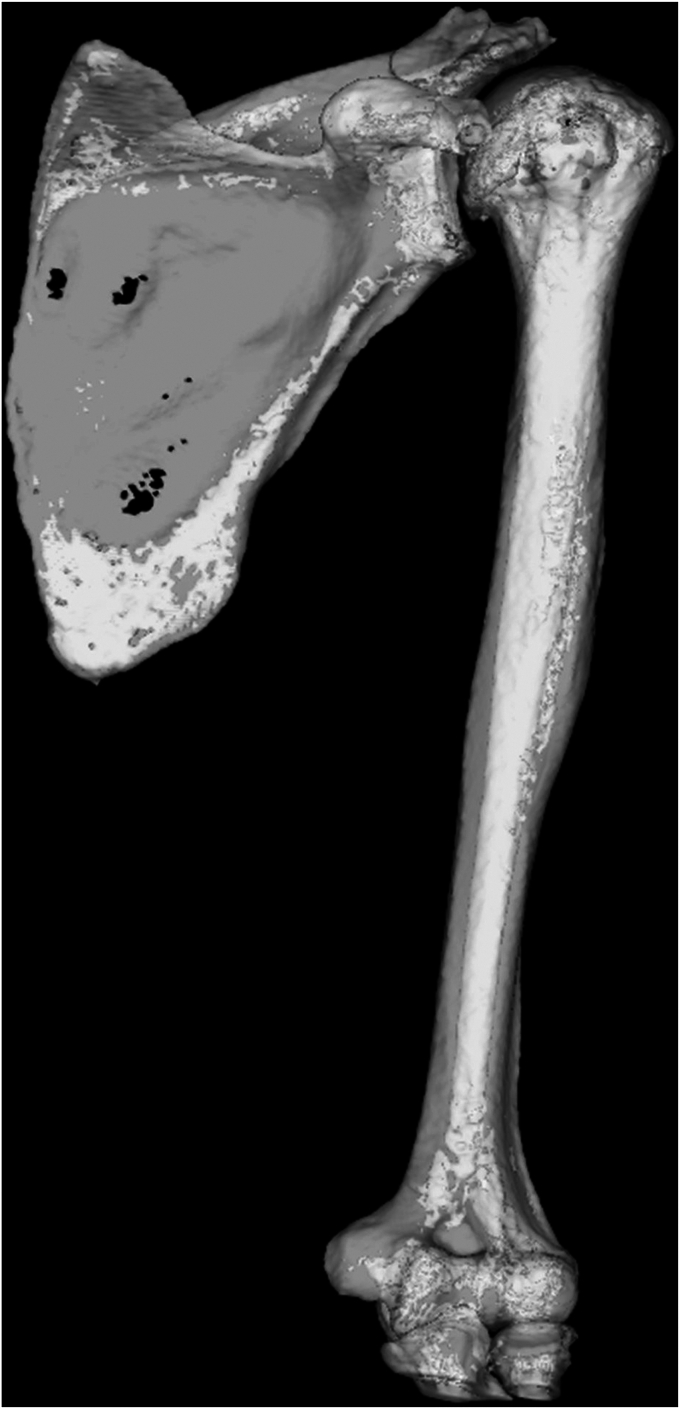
3D bone model created from preoperative computed tomography data of the scapula.
